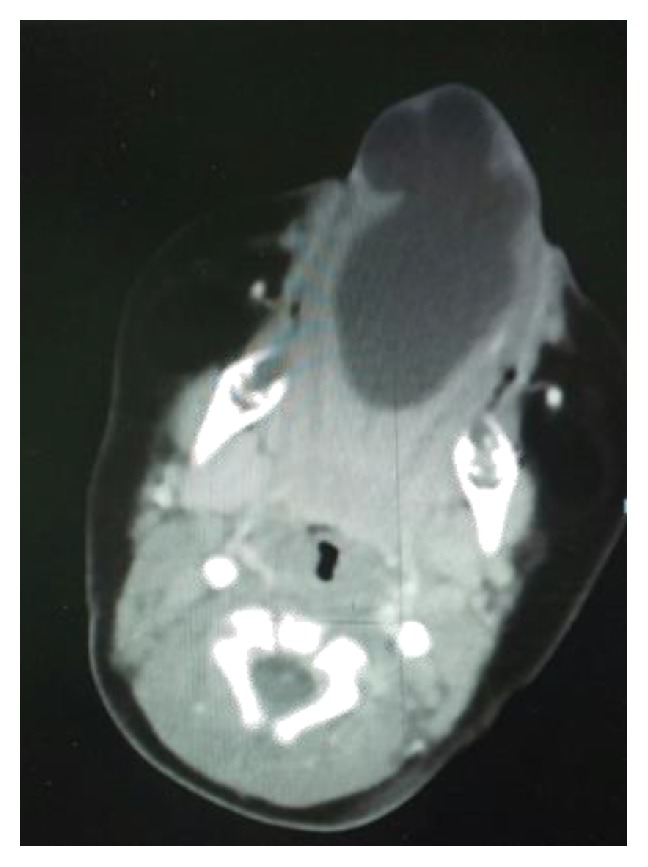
CT neck showing a 4 × 3 × 2 cm cyst in the floor of the mouth.
